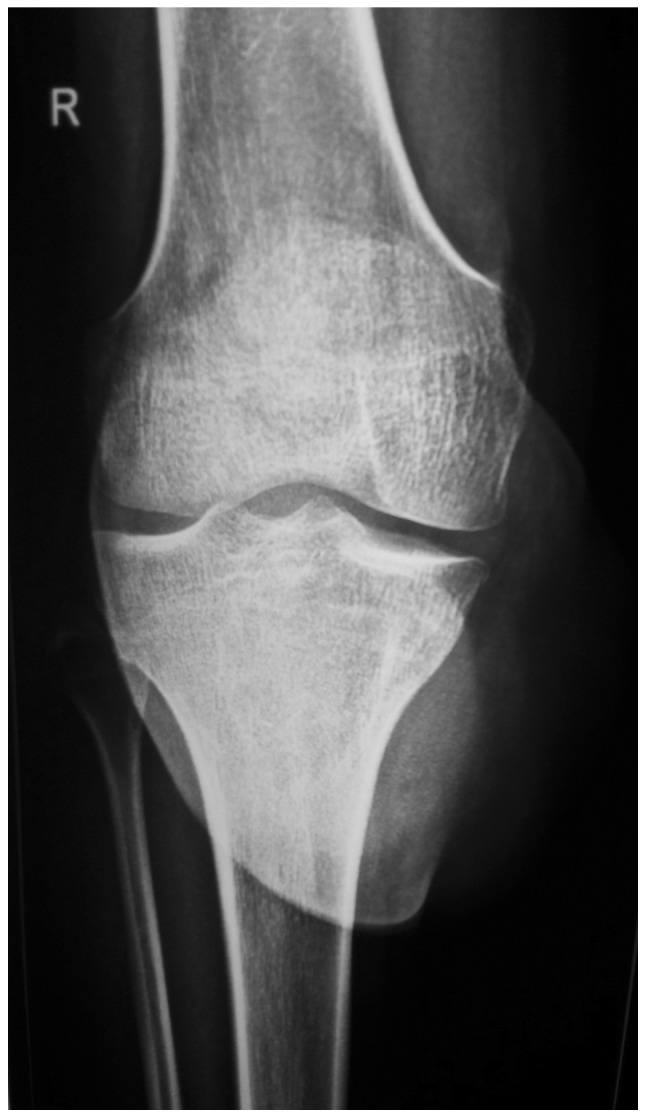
Anteroposterior view X-ray showing a normal joint space and a soft tissue shadow.
